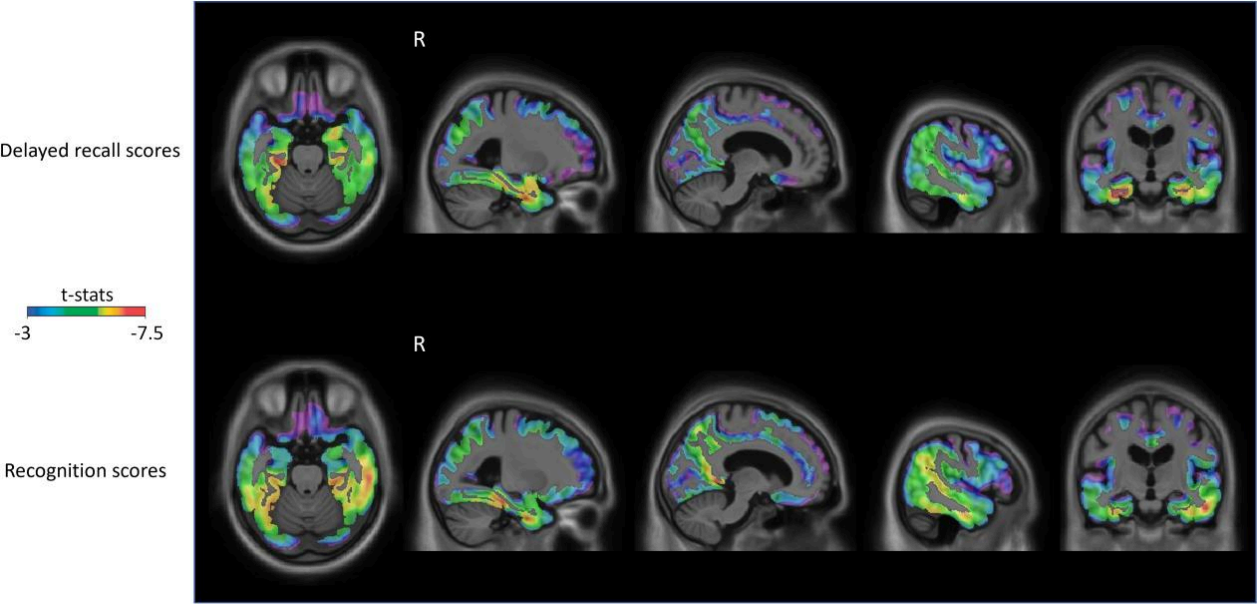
PET scans revealed that delayed recall and recognition scores associate with tau in the same areas, but while delayed recall’s strongest associations with tau arise in antero-mesial and latero-temporal regions, recognition’s strongest associations with tau predominate in posterior temporal and parietal cortices. T-statistical parametric maps were corrected for multiple comparisons using a random field theory cluster threshold of *P* < 0.001, overlaid on the Alzheimer's Disease Neuroimaging Initiative reference template. Two linear regression models were used, were either delayed recall z-scores or recognition d′ scores were entered as outcome variables. Age, sex, years of education, *APOE* status and amyloid-β SUVR were used as covariates.
